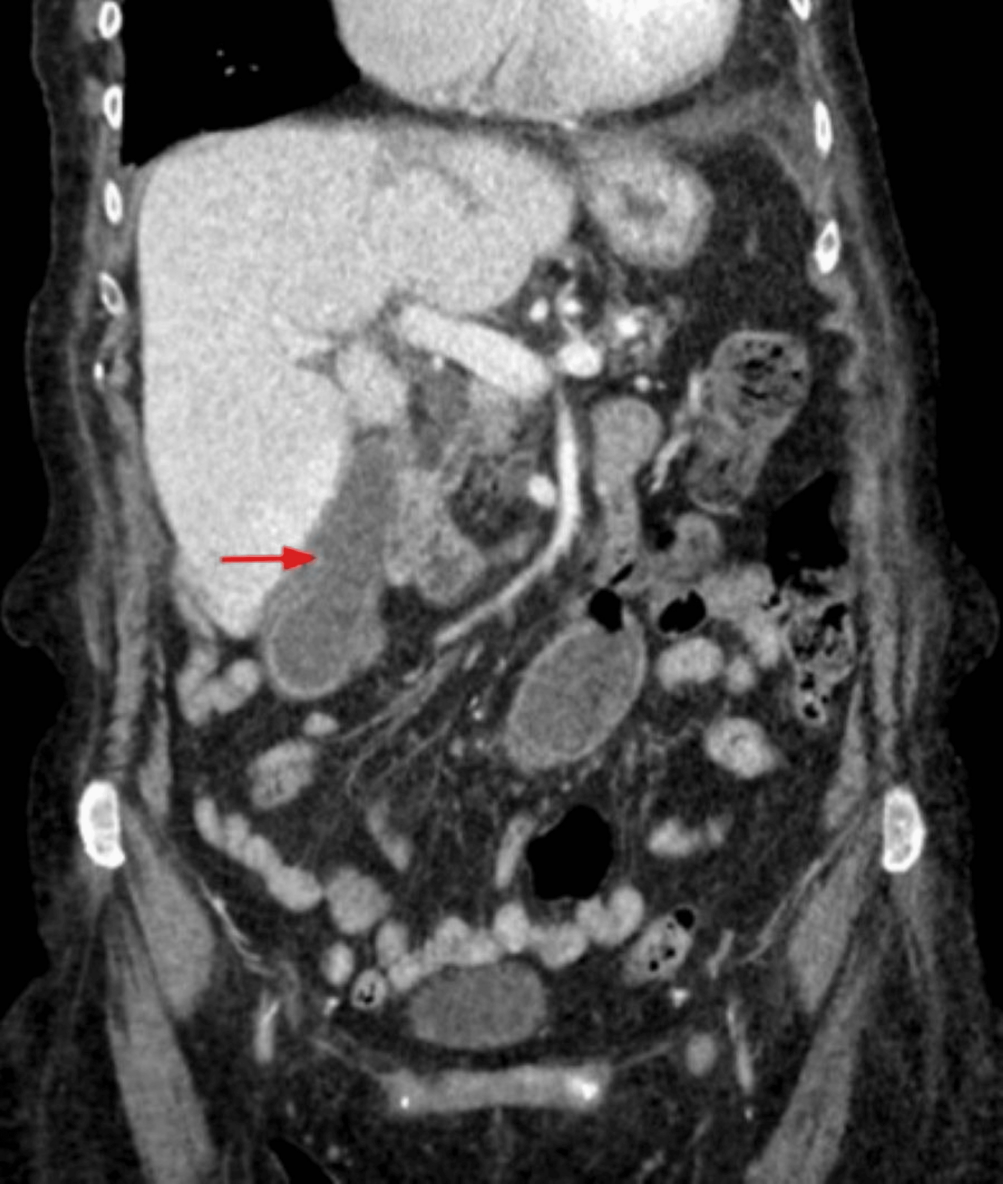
CT abdomen and pelvis with contrast showing a moderately distended gallbladder with mild wall enhancement and pericholecystic inflammatory changes (red arrow). Acute cholecystitis cannot be excluded.
